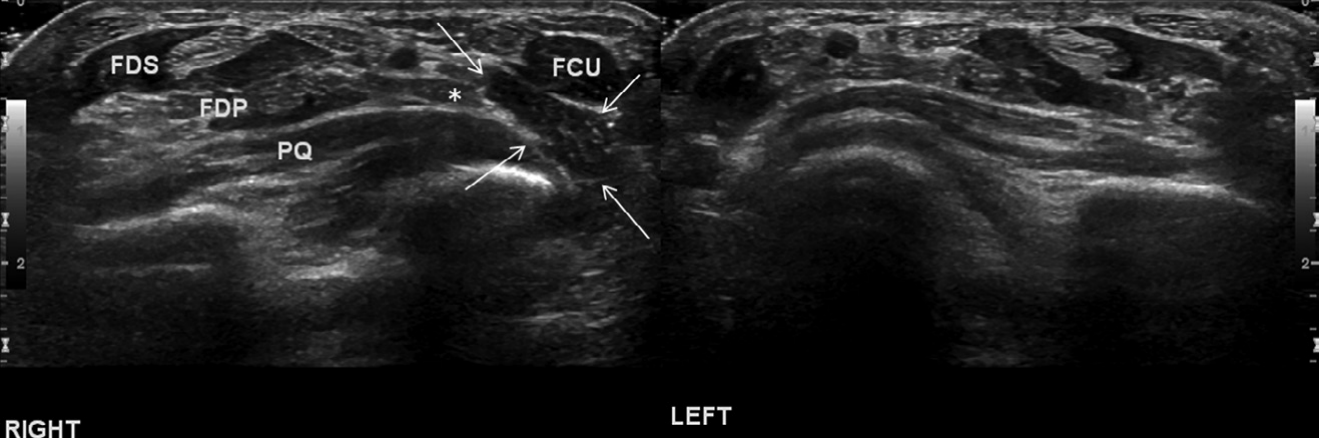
Transverse ultrasound images for “mirror“ comparison of bilateral distal forearms at the level of pronator quadratus muscle demonstrating the accessory muscle bulk deep and separate to normal FCU (white arrows). Note the ulna neurovascular bundle deep to the accessory muscle (asterisk). FCU, flexor carpiulnaris; FDSI, flexor digitorum superficialis.
